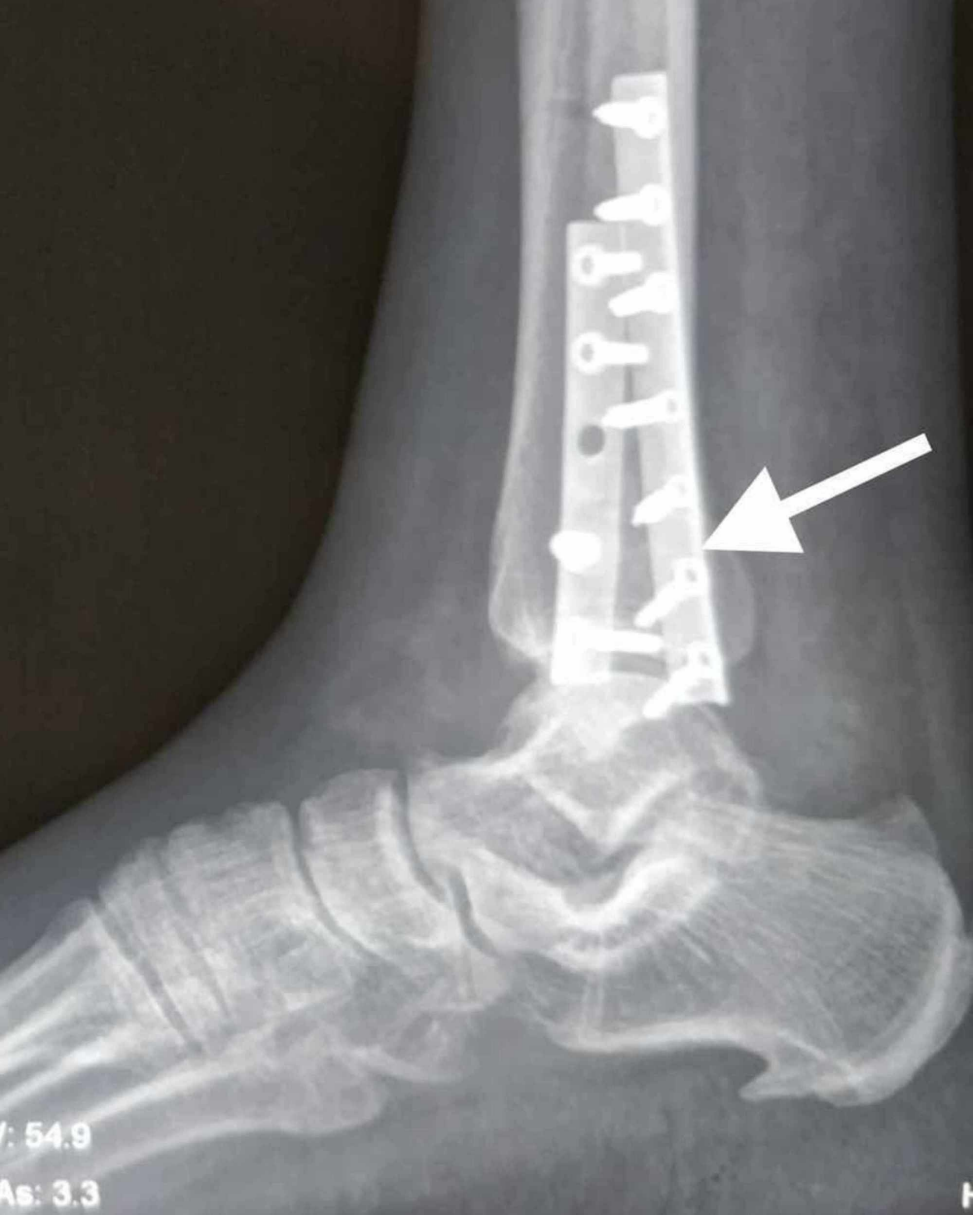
X-ray radiograph lateral view showing the dual plating construct in an osteoporotic distal fibula fracture Note one plate applied on the lateral aspect of the fibula and the other on the posterior aspect of the fibula.
